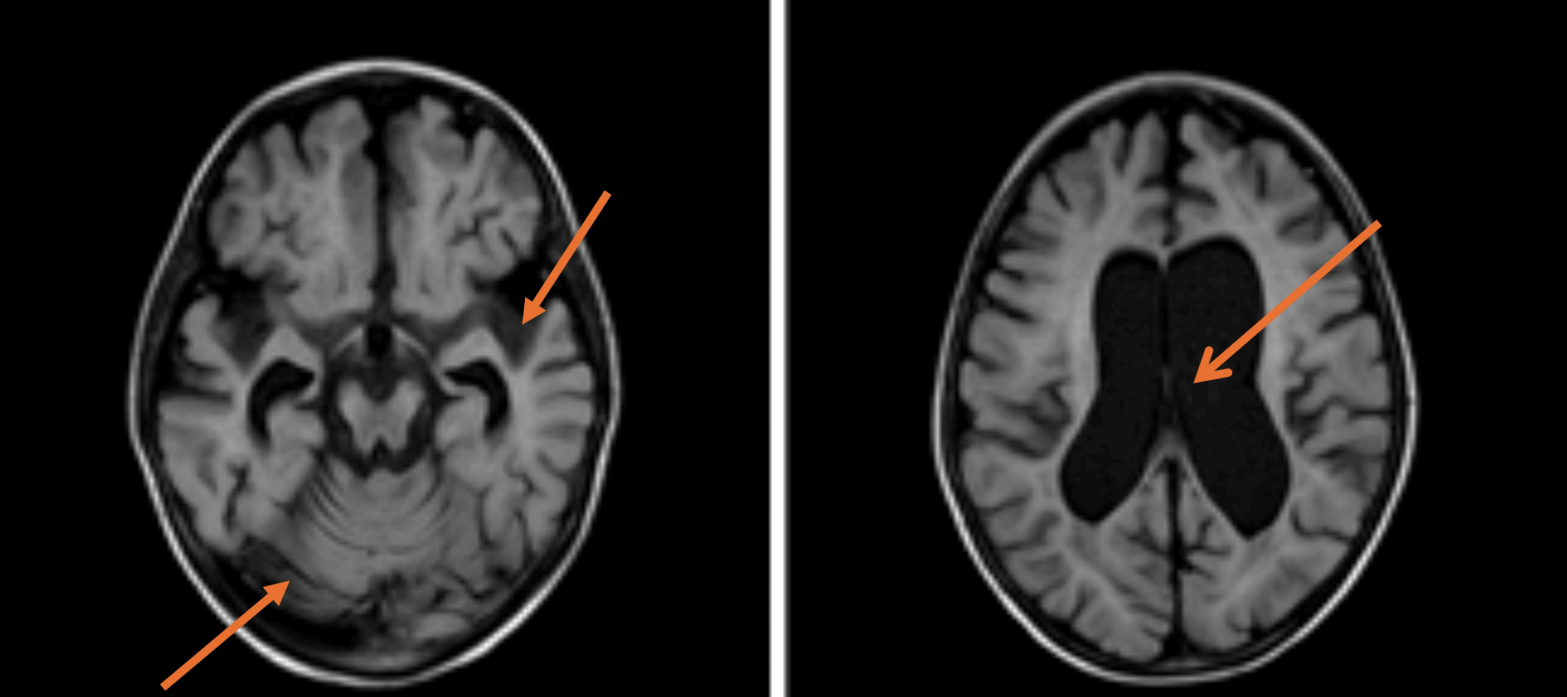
August 2023 cranial MRI The MRI reveals progression in adrenoleukodystrophy, with increased white matter signal alterations in the frontal lobes, corpus callosum, and parieto-occipital regions. Diffuse brain atrophy is indicated by ventricular enlargement, (orange arrows) widened cortical sulci (orange arrows). Loes score remains at 22
